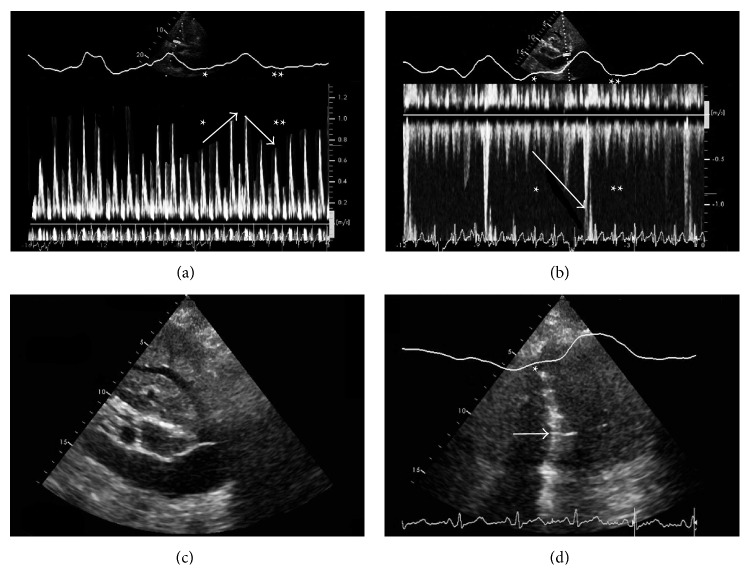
Echocardiographic features of constrictive pericarditis. (a) Pulse wave Doppler recording of tricuspid inflow in apical four-chamber view: increased early diastolic filling velocity during inspiration (ascending arrow), opposite during expiration (descending arrow). (b) Pulse wave Doppler recording of hepatic vein inflow in subcostal view: increased velocity during inspiration (descending arrow). (c) Dilated inferior vena cava and hepatic veins with restricted respiratory variation in subcostal view. (d) Abnormal interventricular septum motion (septal bounce) as a sign of ventricular interdependence in apical four-chamber view: septal bounce to the left ventricle during inspiration (arrow).
